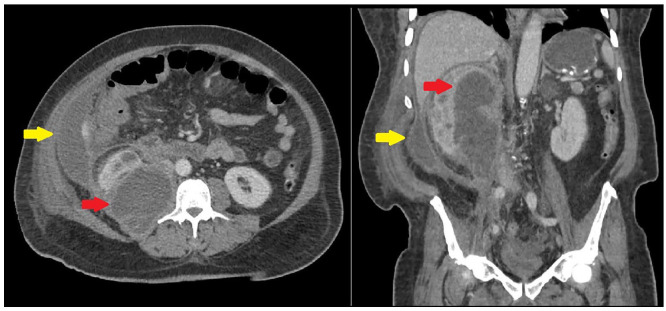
Initial CT showing renal (red arrow) and perihepatic abscesses (yellow
